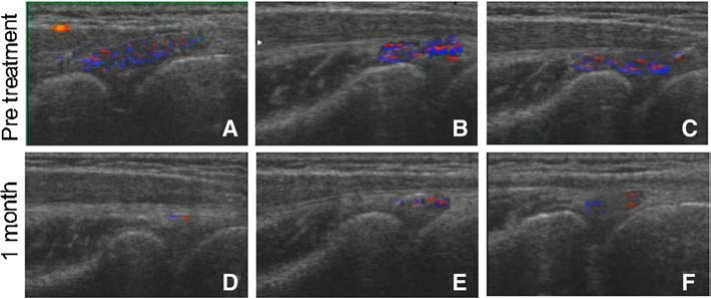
Ultrasonogram graphs of three representative cases before APL injection (a–c) and 1 month after APL treatment (d–f). The areas of color Doppler activity were much smaller at 1 month after APL treatment than those before injection in three patients
